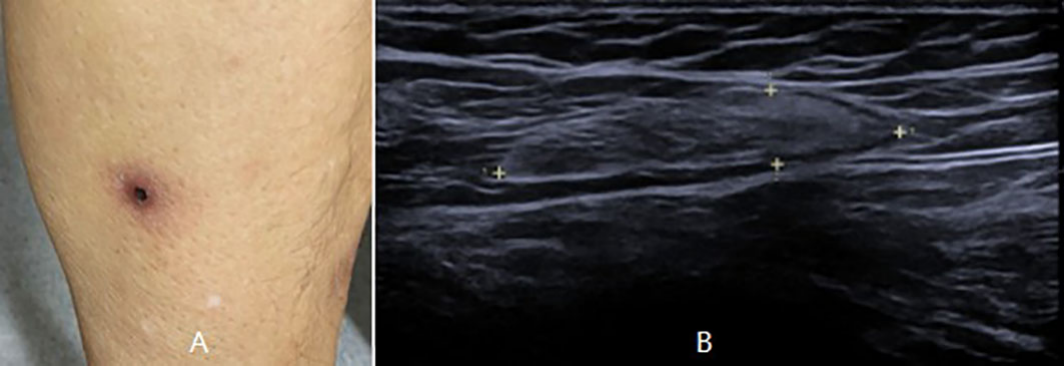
Physical examination showed an eschar on the left leg (A), and B-type ultrasound showed enlarged lymph nodes in the left groin (B).
